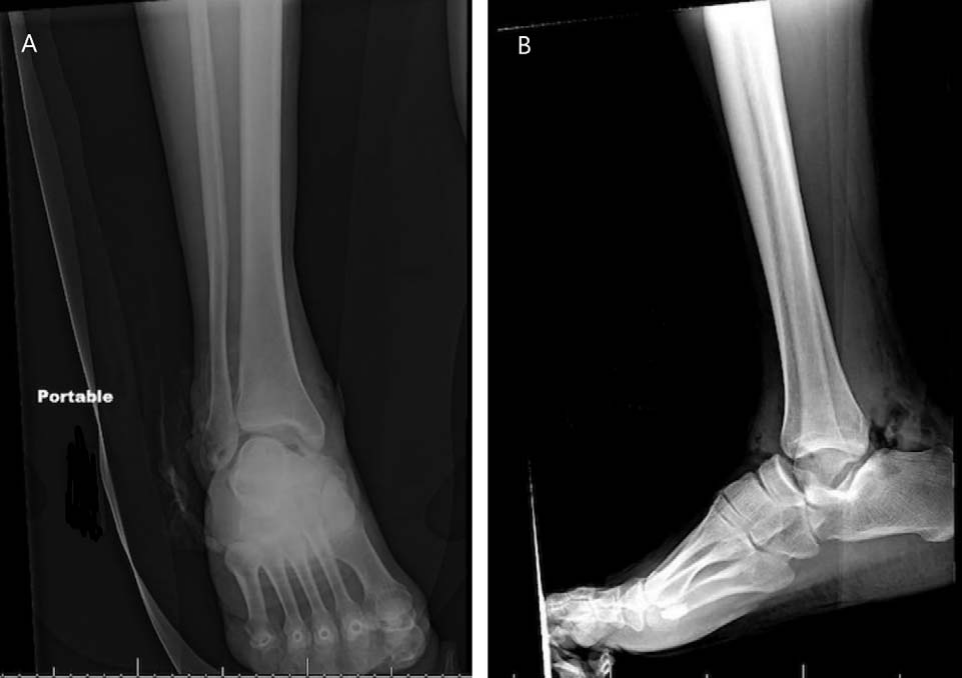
(A) Anteroposterior and (B) lateral emergency department x-rays of the patient's right ankle show complete talar extrusion with soft tissue gas secondary to an open talar extrusion.
